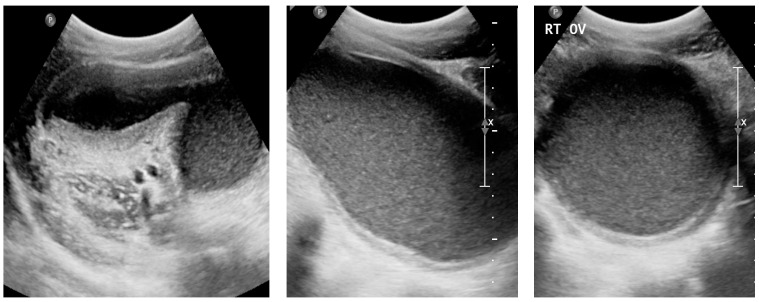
Pelvic ultrasound in a 13-year-old patient (Case 3) showing a markedly distended fluid-filled vagina consistent with hematocolpos secondary to obstructed right hemivagina.
